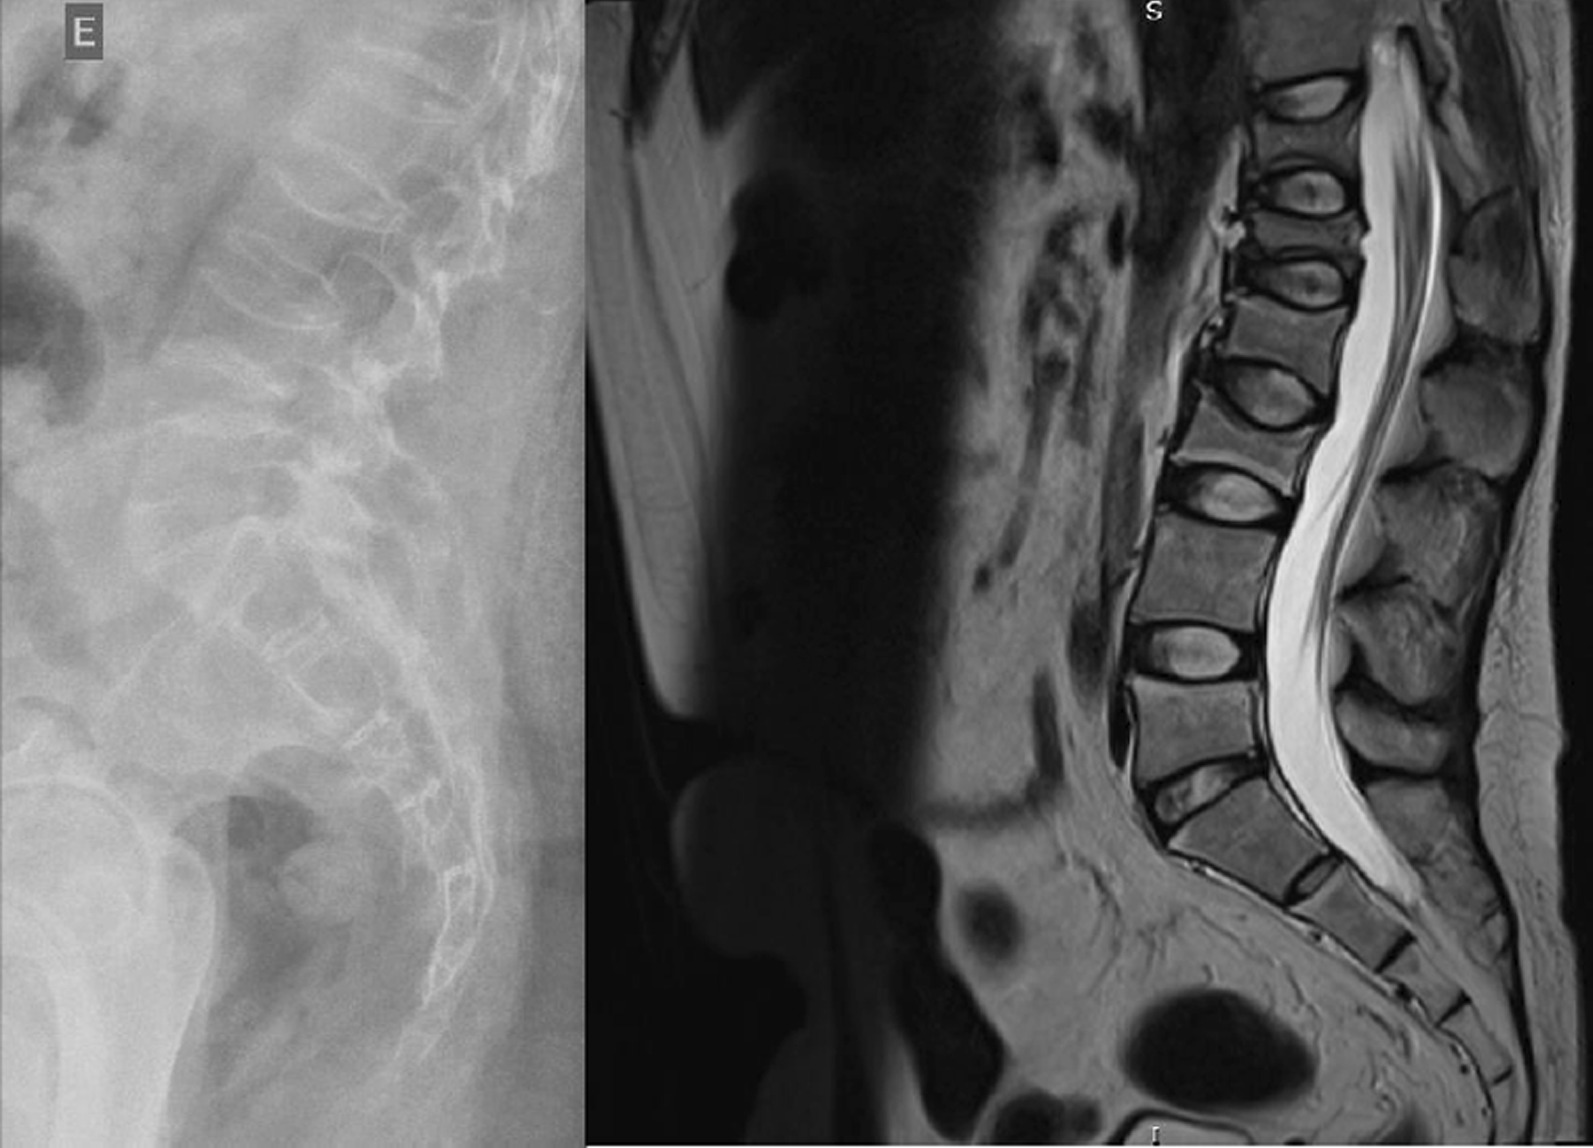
Radiography and Magnetic Resonance Imaging (MRI) of lumbosacral spine in profile
